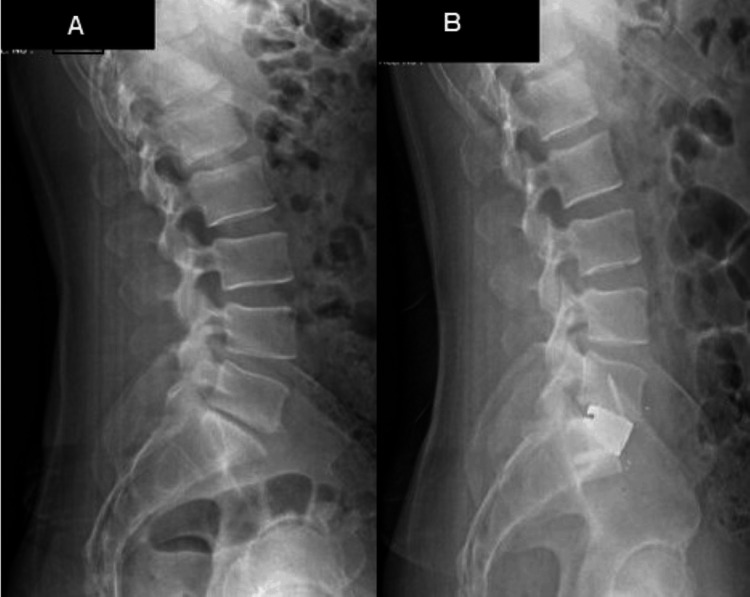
Preoperative and postoperative radiographs after ALIF using the modular cage Preoperative (A) and postoperative (B) radiographic images of a patient with a modular cage at L5-S1. The disc space height increased from 5.3 mm preoperatively to 13.9 mm at 4 days postoperatively using an 8 mm core and measured 13.5 mm at 6-month follow-up. With respect to segmental lordosis, the preoperative Cobb angle was 14.8°, which increased to 26.9° at the early postoperative visit following insertion of the 20° core. The angle measured at the six-month follow-up was 26.0°. ALIF: Anterior lumbar interbody fusion.
